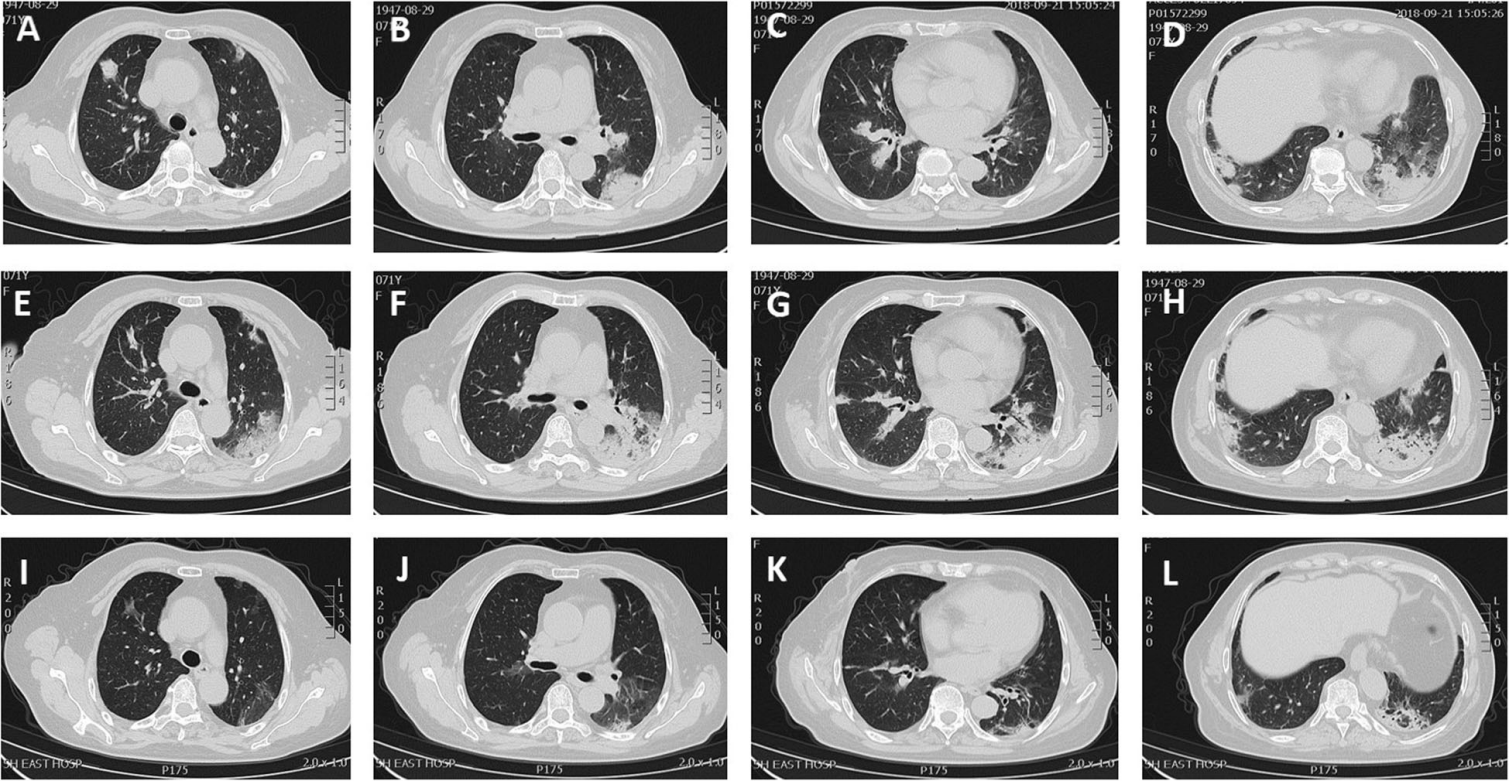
In case 2, CT scan (a, b, c and d) showed geographic and patchy areas of ground glass opacities with interlobular septal thickening, predominantly in basal portions of the lungs. Repeat CT scan (e, f, g and h) after treatment with antibiotics showed diffuse bilateral ground glass opacities. CT images (i, j, k and l) obtained 1.5 months after the initial study demonstrated clearing of diffuse opacities, with residual peribronchial fibrosis and distortion
